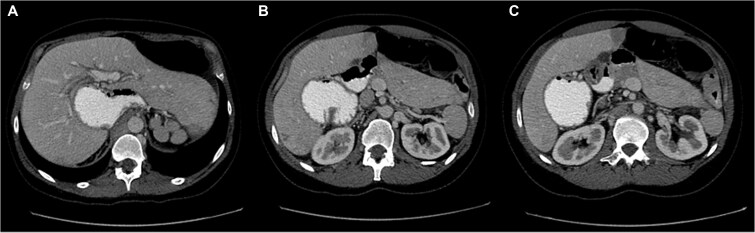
Sequential axial CT images following oral Gastrografin administration showing marked gastric distension and mesenteric vascular swirling, suggestive of volvulus in the setting of intestinal malrotation (A–C).
